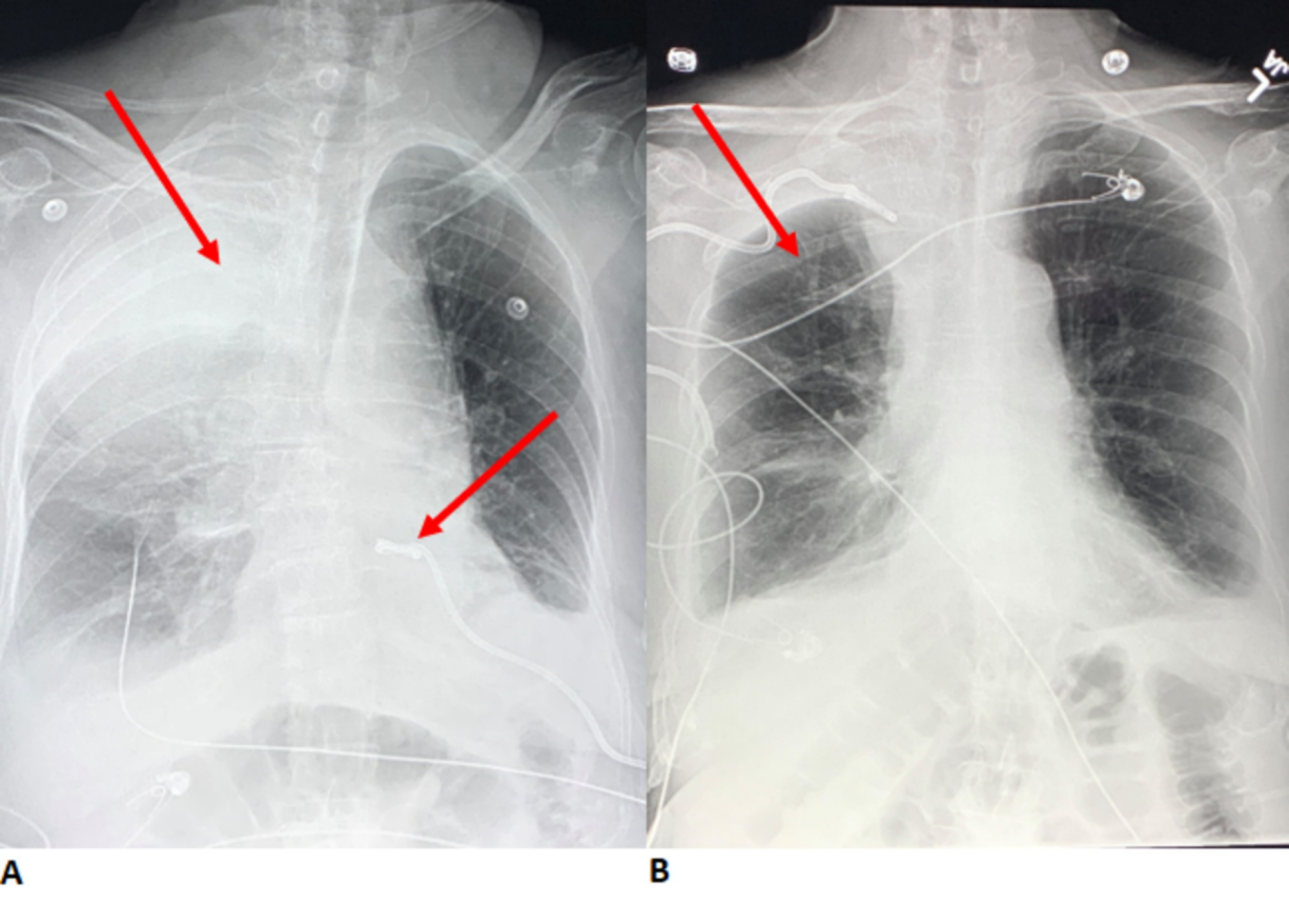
(A) AP chest radiograph with the arrow at the top pointing to a density located near the apex of the lung, consistent with a positive apical cap sign. The arrow at the bottom points to the previously placed mediastinal drain. (B) AP chest radiograph taken after CT-guided drainage of the extrapleural hematoma. AP, anteroposterior.
